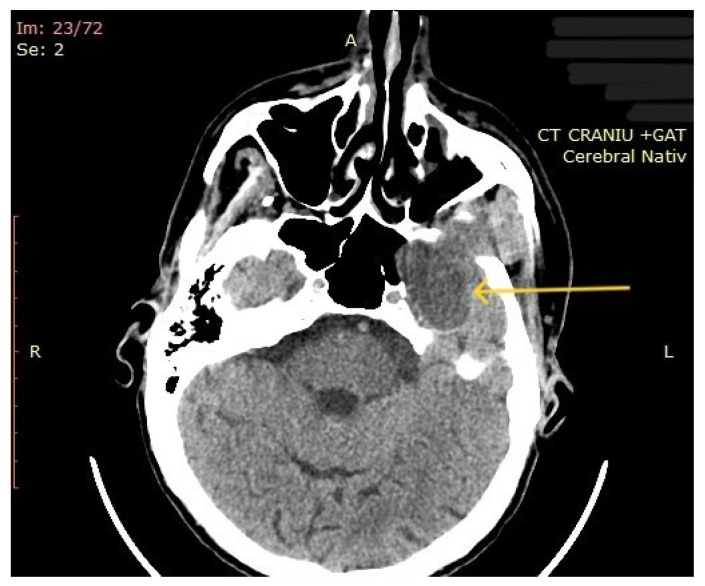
CT imaging: intracerebral component of the mass (the arrow represents the tumor mass).
